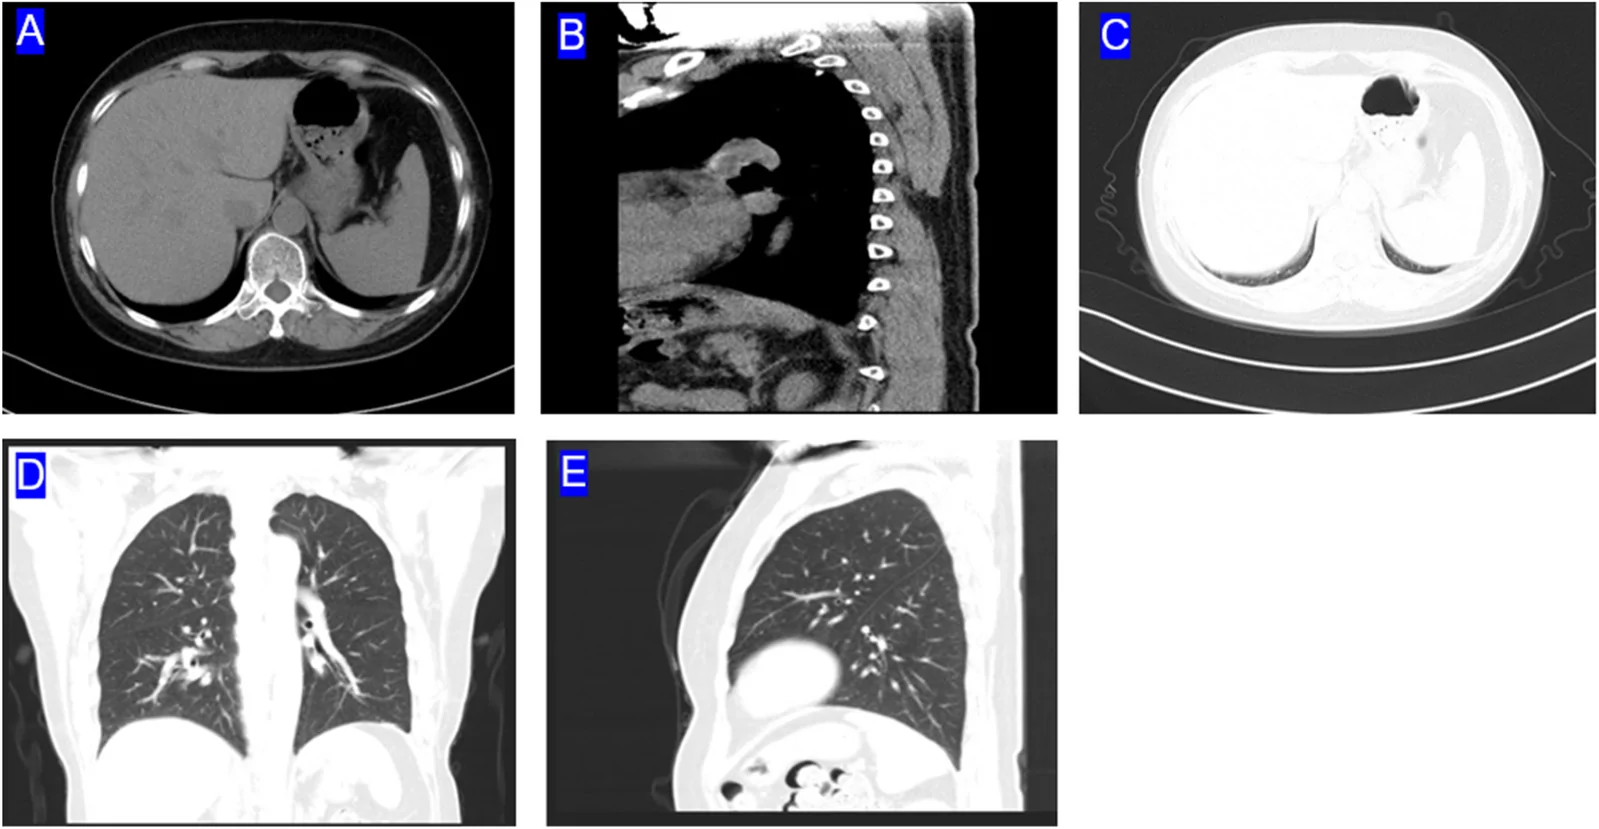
Three-month follow-up chest CT images (A–E) in sagittal, coronal, and axial views, demonstrating complete repair of the diaphragmatic hernia with no recurrence and full re-expansion of the left lung.
